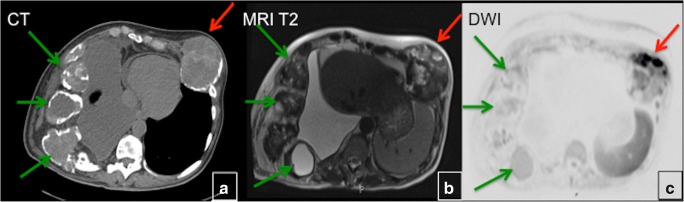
The significance of MRI with diffusion-weighted imaging (DWI) in the patient with polyostotic fibrous dysplasia (FD). CT (a), T2-weighted MRI (b) and DWI (c) show multiple rib lesions (*green arrows*). The left rib lesion (*red arrow*) demonstrates restricted diffusion, which requires further evaluation to rule out malignant transformation
